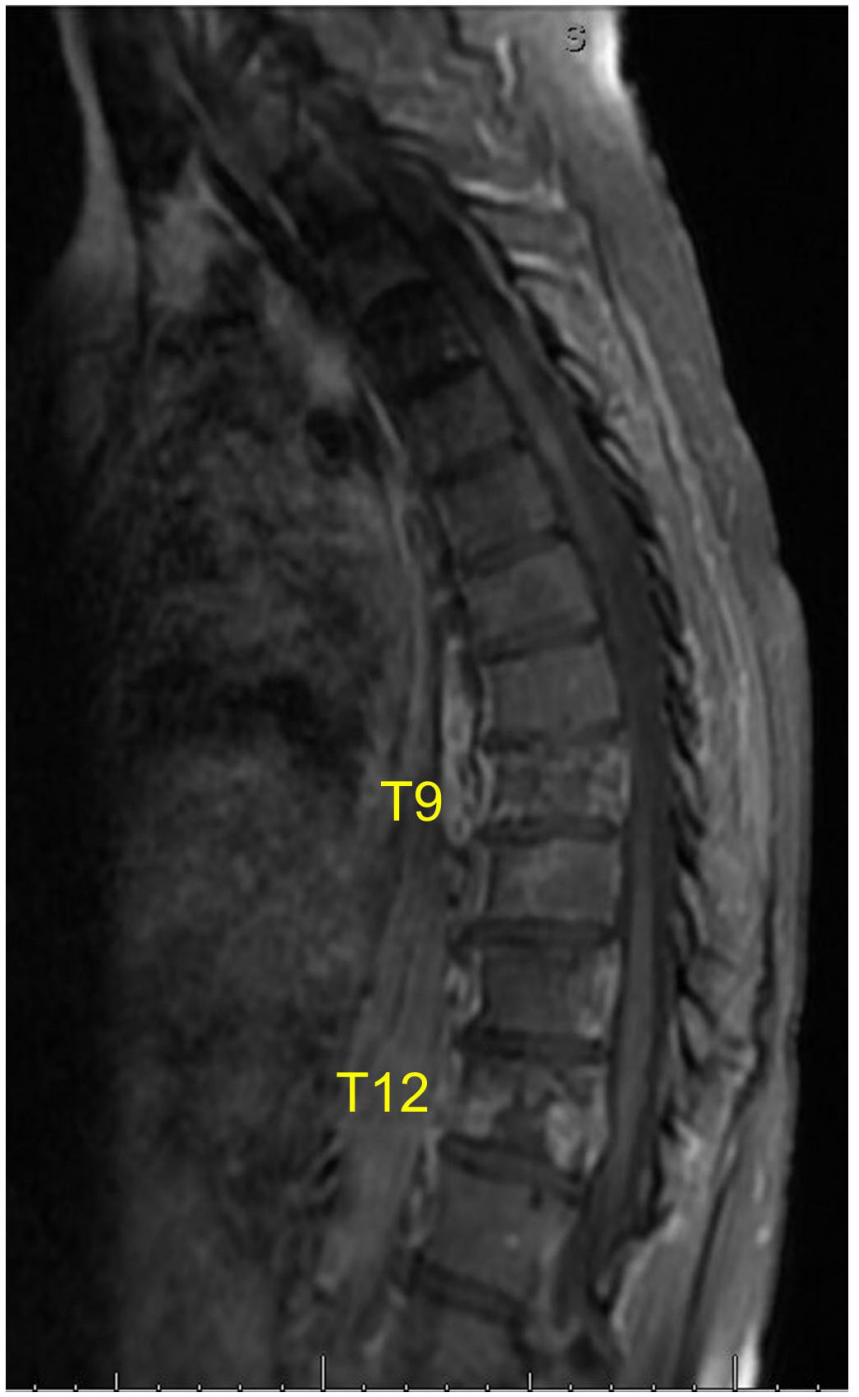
Preoperative sagittal MRI (T2-weighted) shows lesions at the T9 and T12 vertebral bodies.
